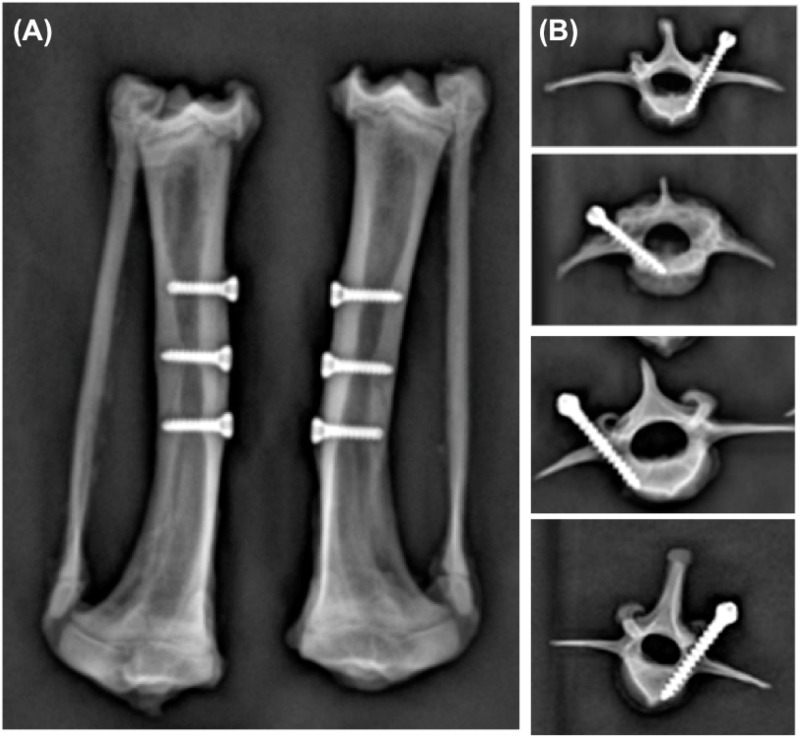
Representative radiographs showing cortex and pedicle screws after implantation. (A) Cortex screw with cortical bone placement and (B) Pedicle screw with traditional placement on L2-L5 vertebrae.
